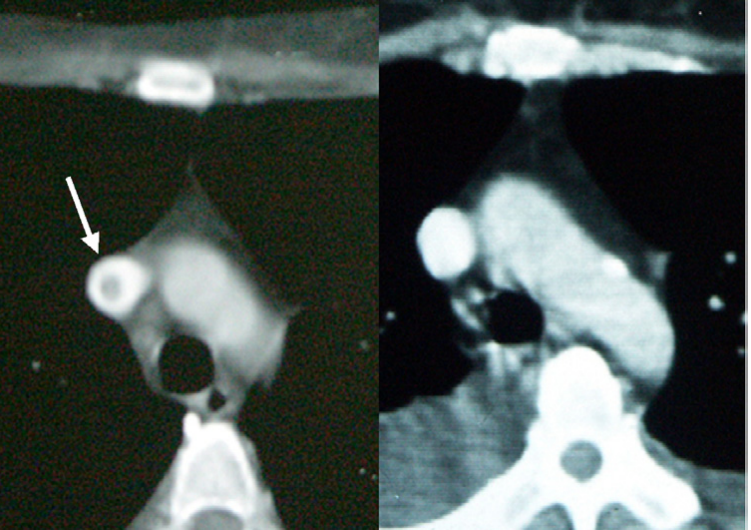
CT scan showing thrombus before and after thrombolysis. 1a. CT scan of superior vena cava with intravenous contrast infusion showing a thrombus before streptokinase infusion. 1b. CT scan of superior vena cava with intravenous contrast infusion three days after thrombolysis showing no remaining thrombus.
